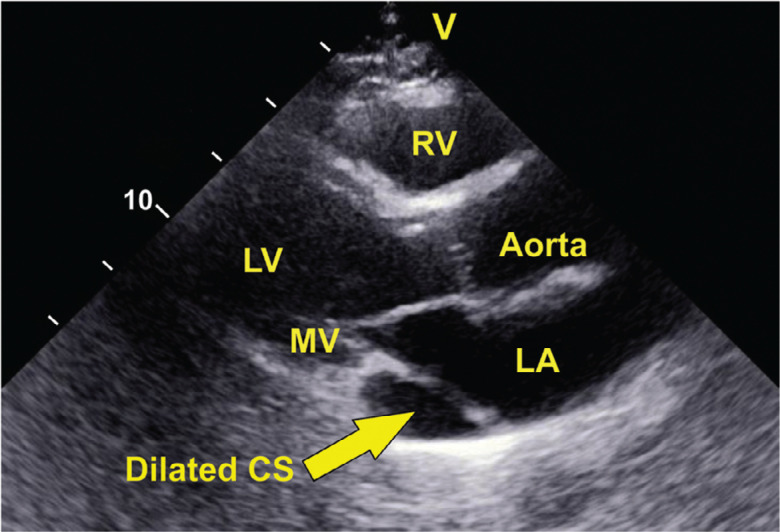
Transthoracic echocardiography in the parasternal long-axis view shows a dilated CS. *Abbreviations:* CS, coronary sinus; LA, left atrium; LV, left ventricle; MV, mitral valve; RV, right ventricle.
